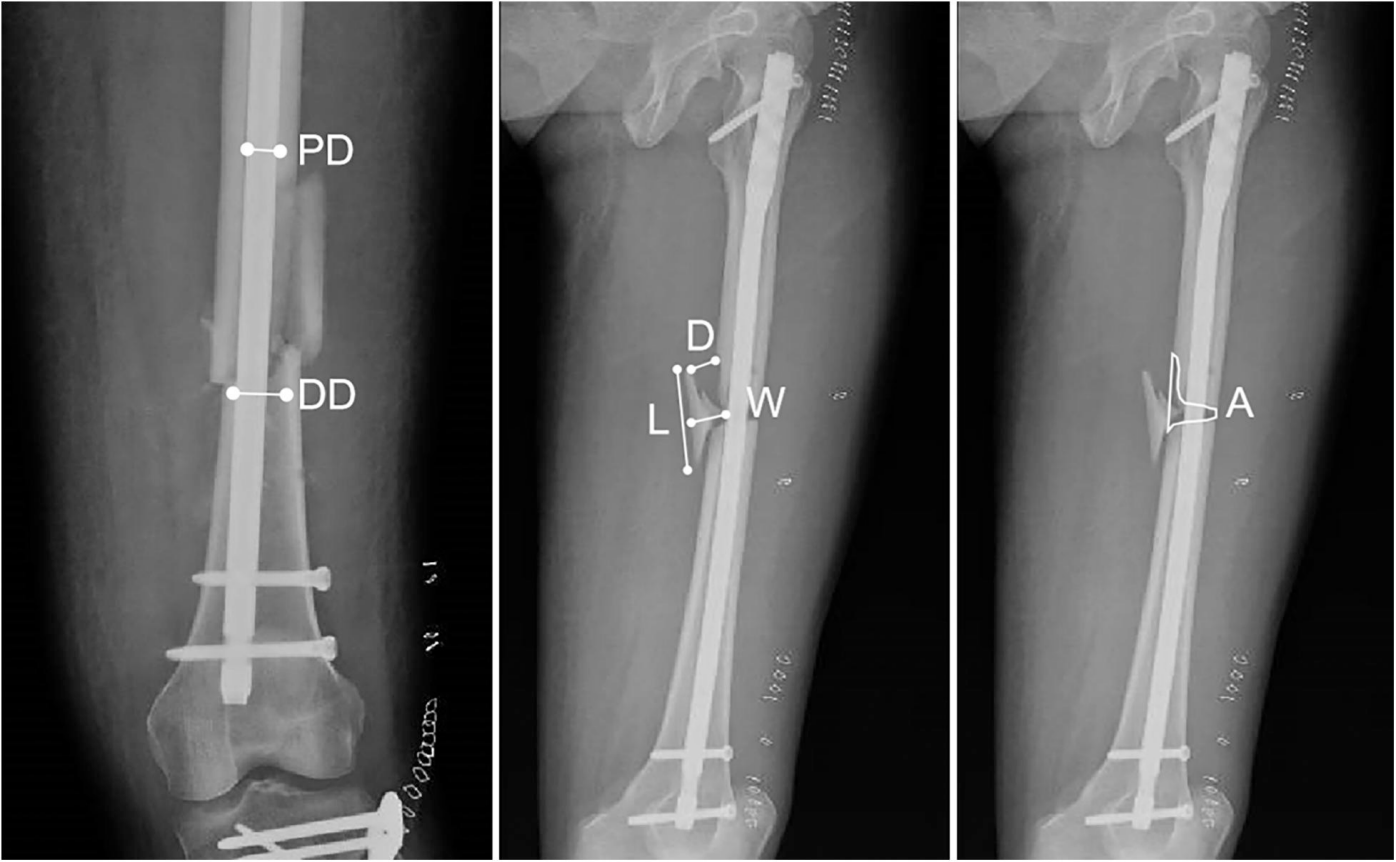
Postoperative radiographic measurements. PD: canal diameter at the proximal end of fracture. DD: canal diameter at the distal end of fracture. D: displacement of fragment. L: length of fragment. W: width of fragment. A: area of the fracture gap.
